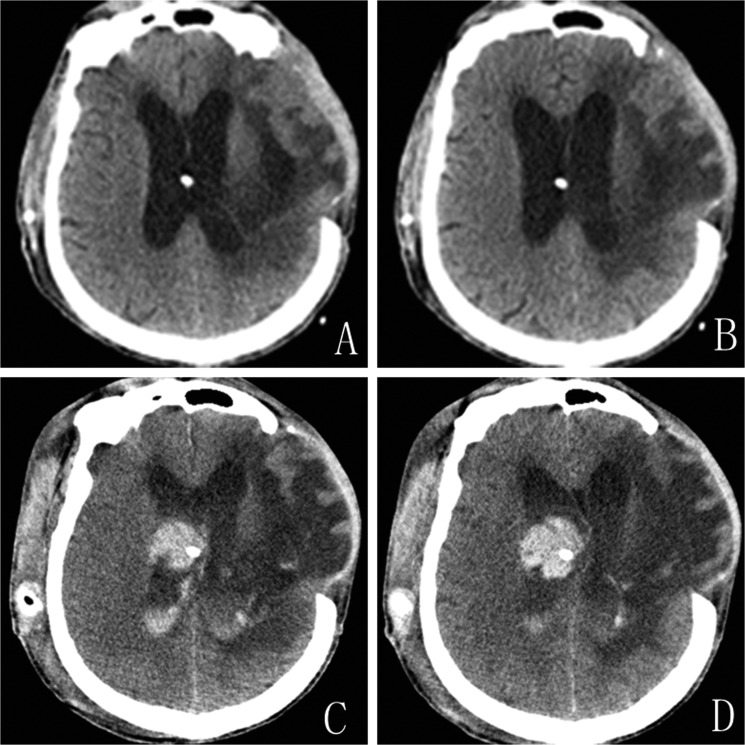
Patient 1. (A and B) CT scans obtained one day after shunt placement demonstrating shrinking ventricles and no hematoma. (C and D) CT on postoperative day three showed intraventricular hemorrhage along the path of ventricular catheter.
